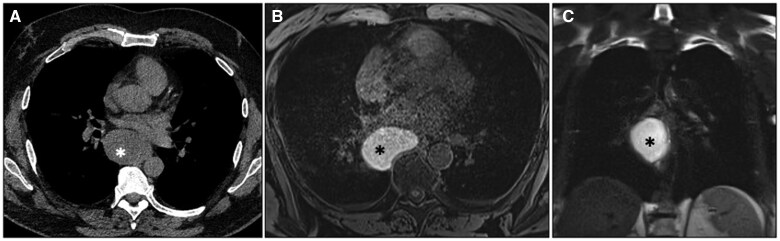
Bronchogenic cyst. (A) Non-contrast CT axial image shows a homogeneous lesion (asterisk) in the right visceral mediastinum under the level of the carina, with attenuation values slightly higher than fluid. (B, C) Axial T1-weighted and coronal T2-weighted MR images show high-signal intensity, consistent with a cystic lesion with a heterogeneous content.
